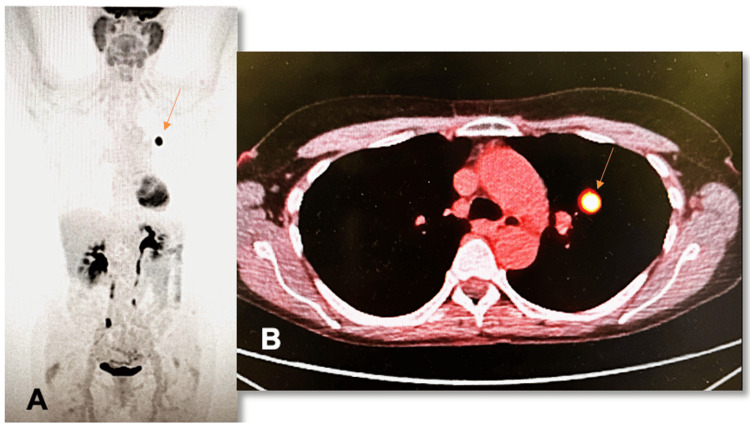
PET/CT scan showing an FDG-avid solid left upper lobe nodule with no evidence of regional lymph node involvement or distant metastatic disease (orange arrow) in (A) coronal and (B) transverse plane. PET/CT: positron emission tomography/computed tomography; FDG: fluorodeoxyglucose
